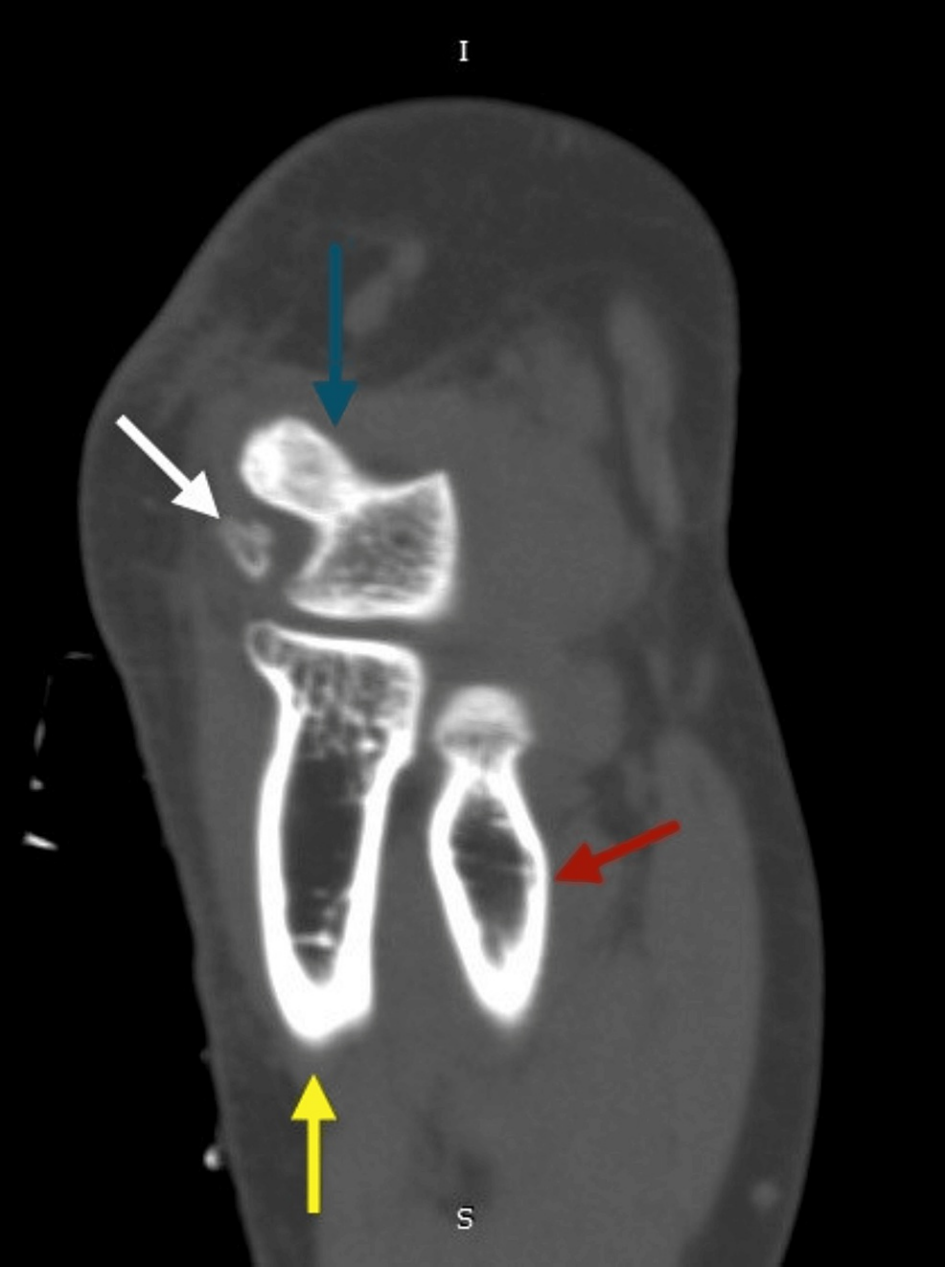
Computed tomography scan (sagittal view) of the left elbow joint The white arrow indicates the os subepicondylare mediale. The blue arrow indicates the medial humeral epicondyle. The red arrow indicates the radius. The yellow arrow indicates the ulna.
